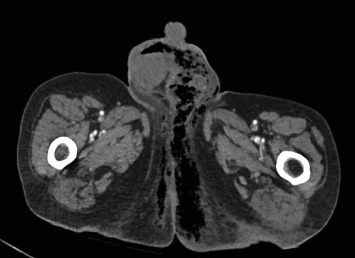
Axial CT image obtained on arrival reveals the presence of air loculi within the perineum and scrotum, consistent with necrotizing soft tissue infection.
